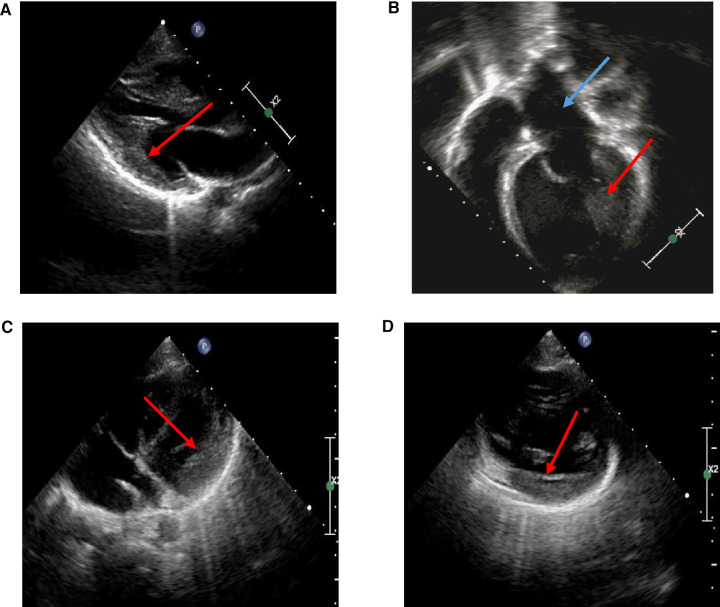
Echocardiography in HCM probands. (A) Echocardiography of a proband: slight left ventricular hypertrophy at later observation (red arrow). (B) Echocardiography of a proband: enlargement of left atrial (blue arrow) and left ventricle (red arrow) at first time. (C,D) Echocardiography of a proband: slight left ventricular hypertrophy (red arrow).
